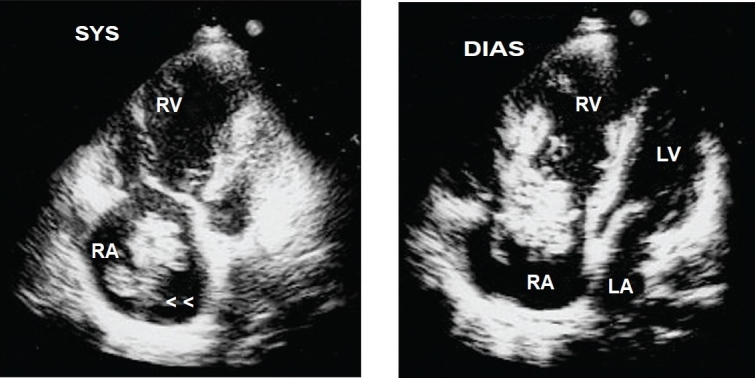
Transthoracic echocardiogram showing a large free-floating thrombus (arrowhead) in the right atrium prolapsing into right ventricle through the tricuspid valve in a patient with polycythemia rubra vera and splanchnic vein thrombosis. Abbreviations: RA=Right atrium; RV=Right ventricle; LA=Left atrium; LV=Left ventricle.
